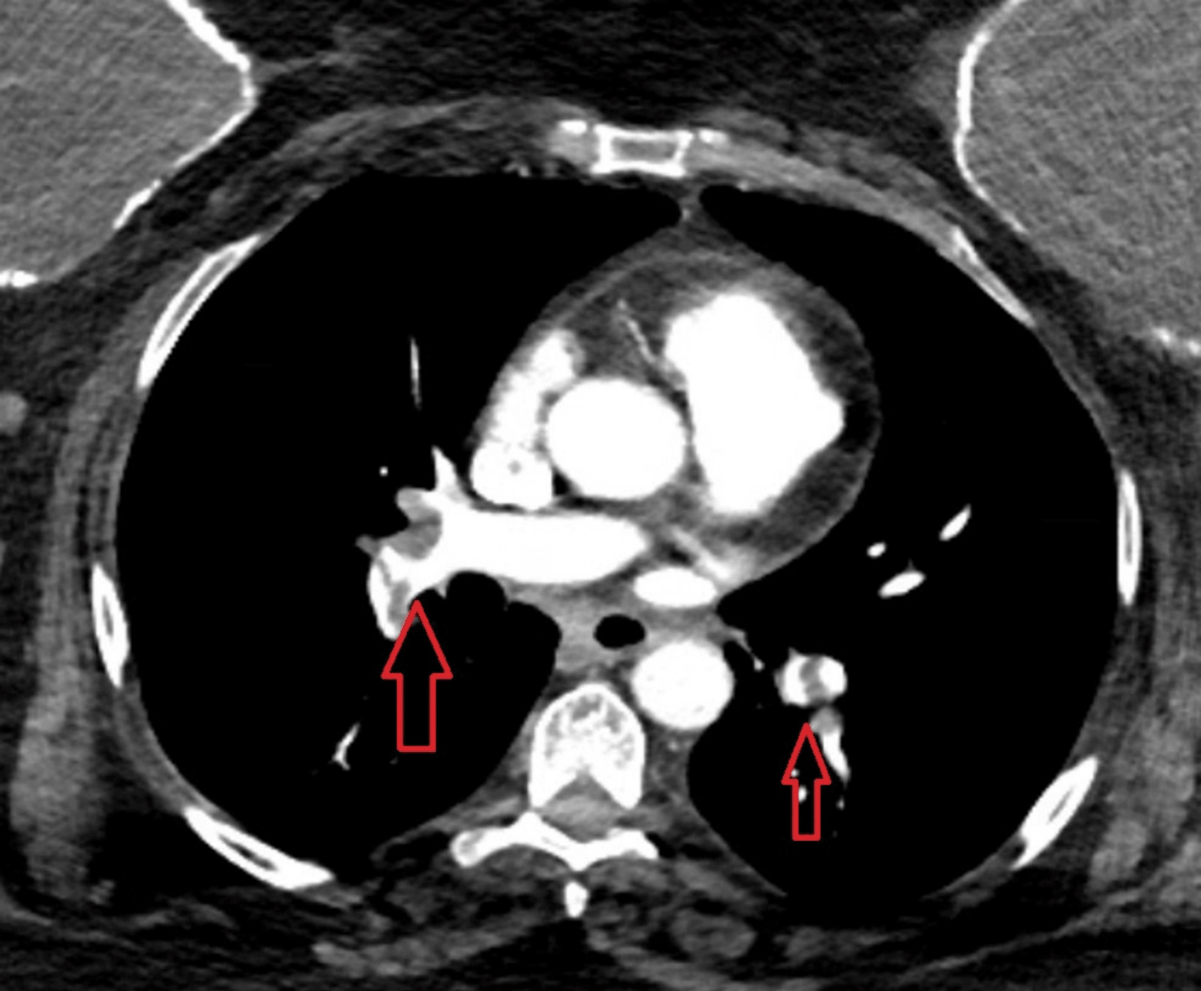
CT angiogram. Notable for bilateral pulmonary emboli (red arrows) with right heart strain
